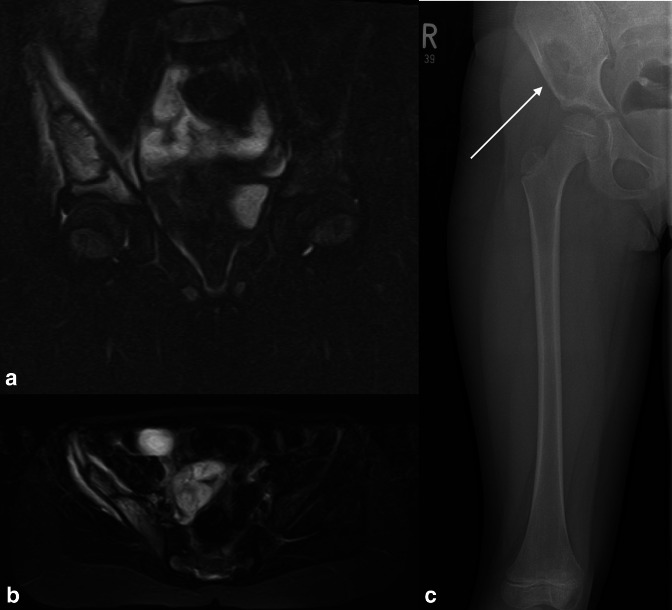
WBMRI in a 6-year-old with langerhans cell histiocytosis. Coronal (A) and axial (B) STIR MRI shows a focal region of well-defined high T2 signal with a peripheral low signal rim in the right iliac wing. There is bone marrow oedema in the ileum and ischium, and surrounding soft tissues. Plain radiography shows a classic corresponding well-defined lytic abnormality without peripheral sclerosis and a narrow zone of transition (C).
